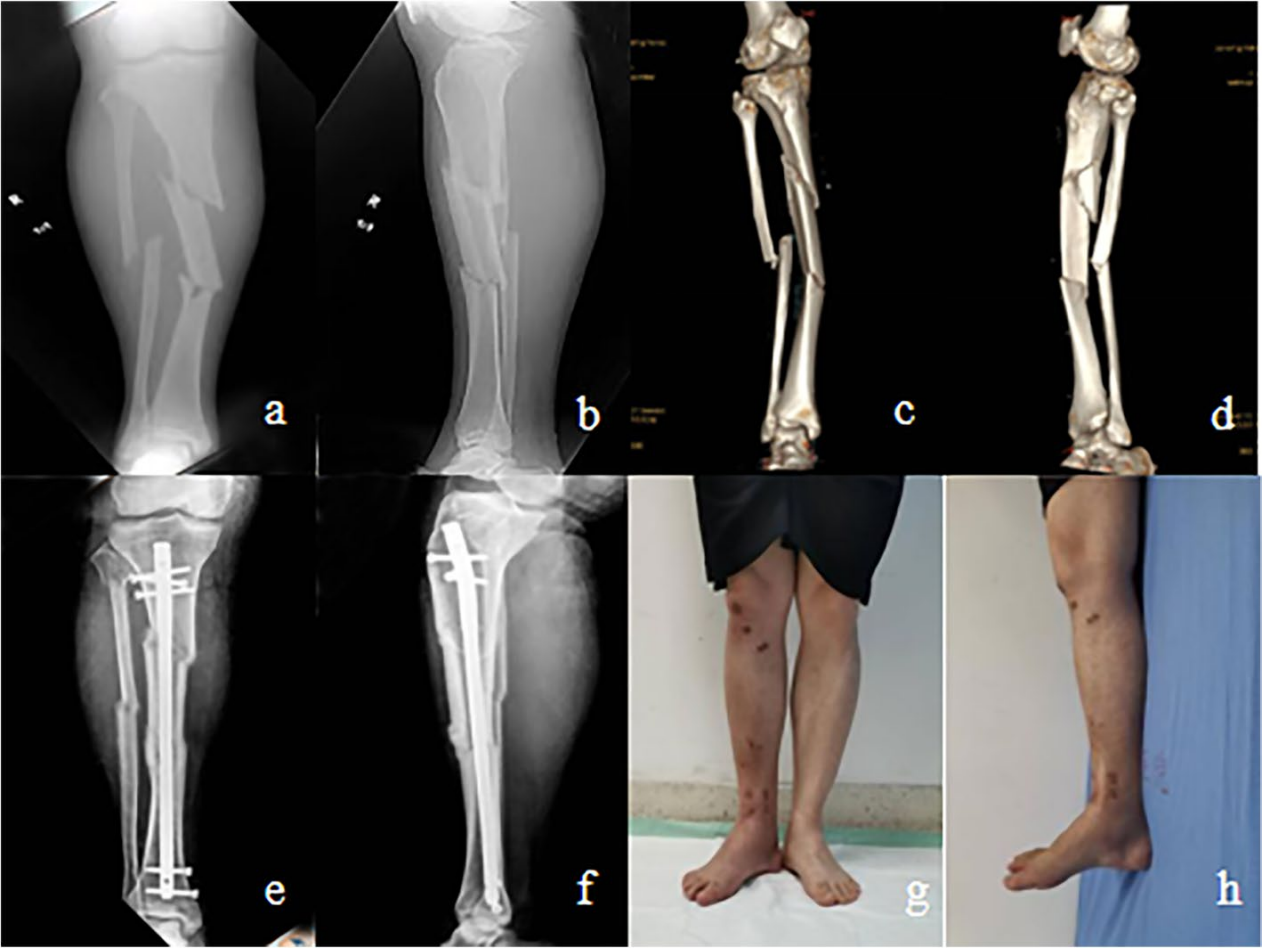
Images of treating a 48-year-old female with STFs due to traffic accident by using IMN. a, b X-ray images of injured STFs preoperatively. c, d Computed tomography reconstruction images of injured STFs preoperatively. e, f Images show good fracture healing 6 months postoperatively. g, h Clinical follow-up appearance images obtained 1 month after IMN removal
